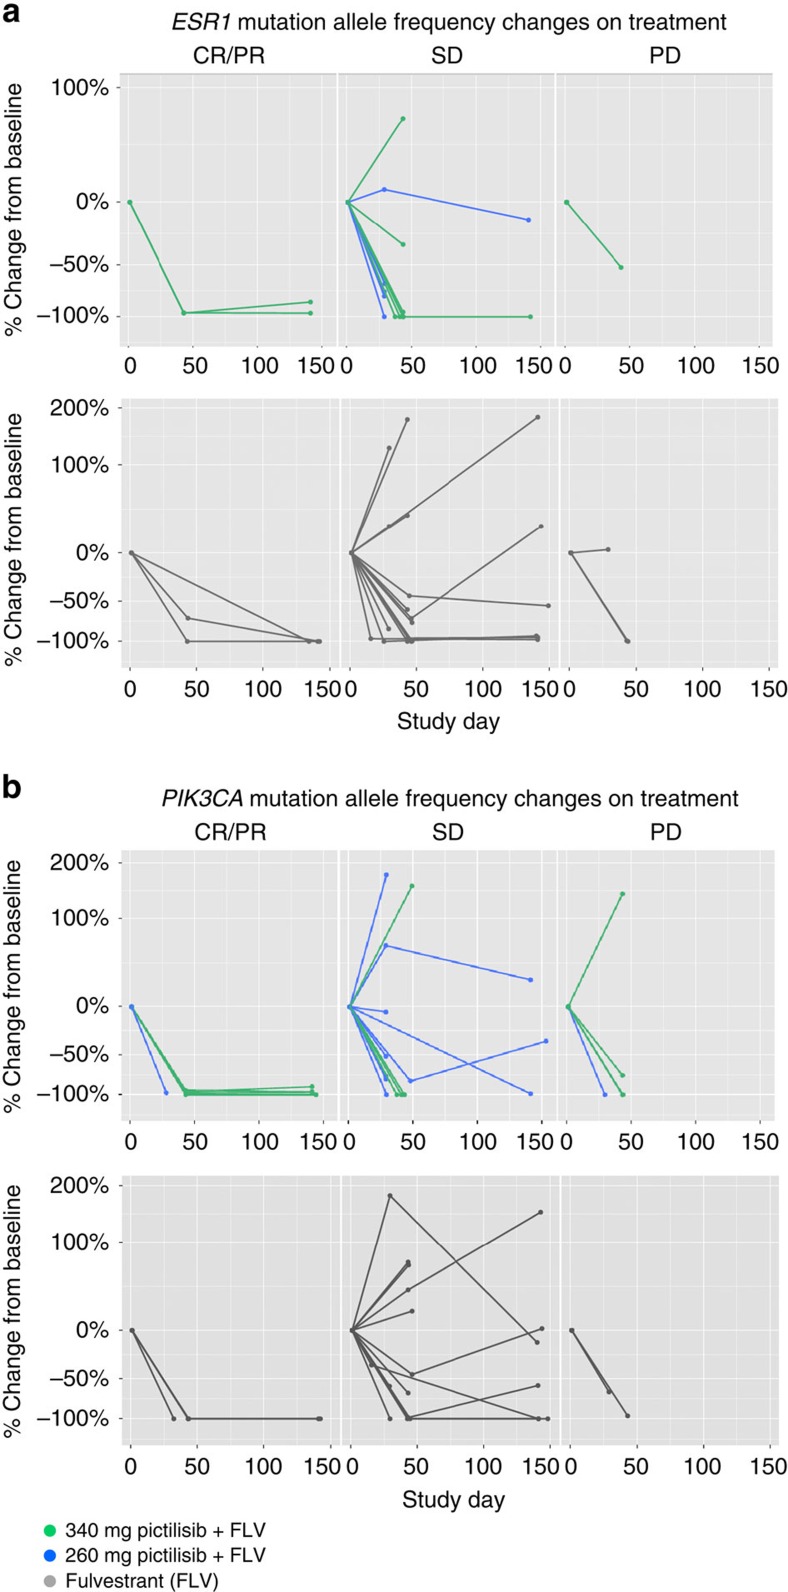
Serial analysis of ctDNA mutation AF changes binned by best clinical response. (a) *ESR1* and (b) *PIK3CA* ctDNA mutation AF changes while on part 1 (green) or part 2 (blue) pictilisib+fulvestrant or placebo+fulvestrant (grey) treatment arms. Patients are binned according to their best clinical response: CR/PR defined by RECIST criteria, and SD or PD as assessed by radiographic scans. Patients with a best response of non-CR/non-PD for patients without measurable disease at baseline are binned with SD. Each line represents one patient with the per cent change of AF relative to baseline (study day 0) plotted for two or three time points. The mutation AF sum is plotted for patients with multiple *ESR1* (a) or *PIK3CA* (b) mutations.
